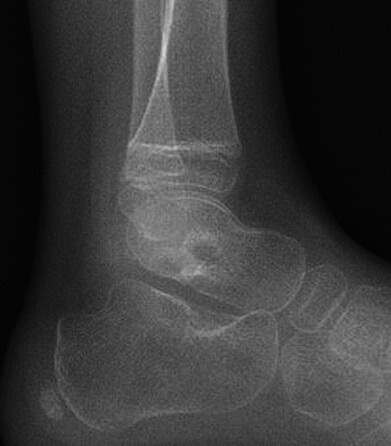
Satisfactory lateral radiograph of the talus showing no signs of osteomyelitis and good bony alignment.
